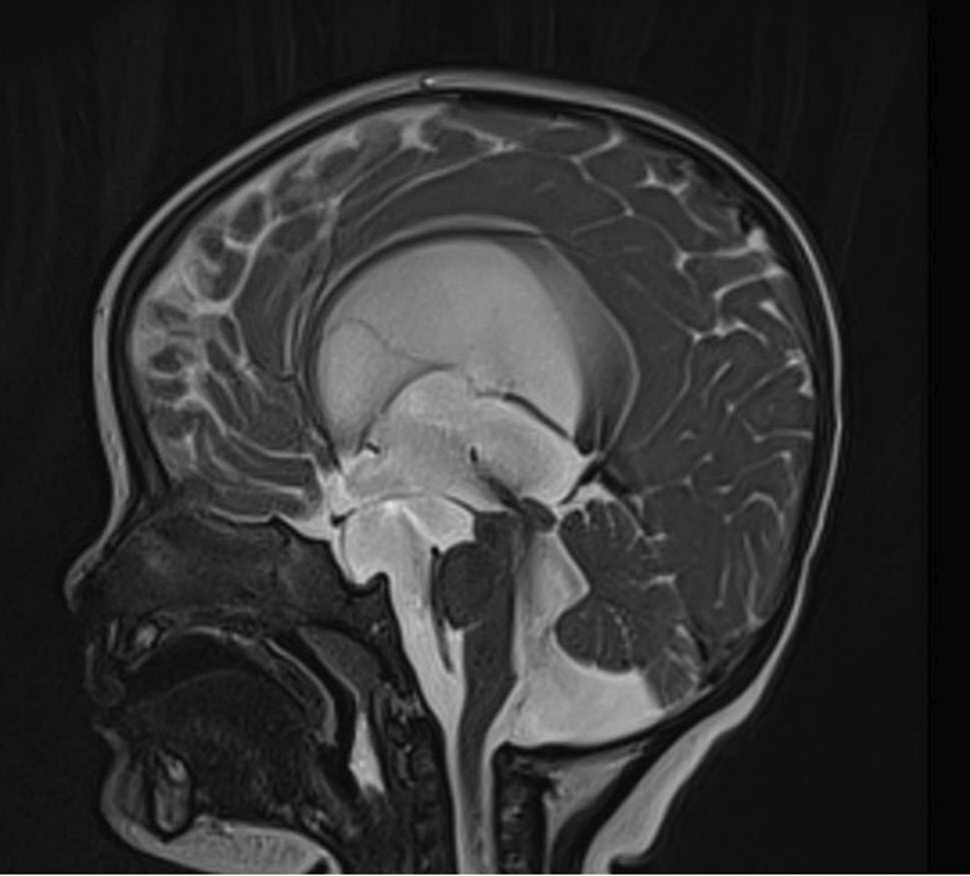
Preoperative MRI of a 1-year-old female showing a suprasellar cyst with a secondary PPB
